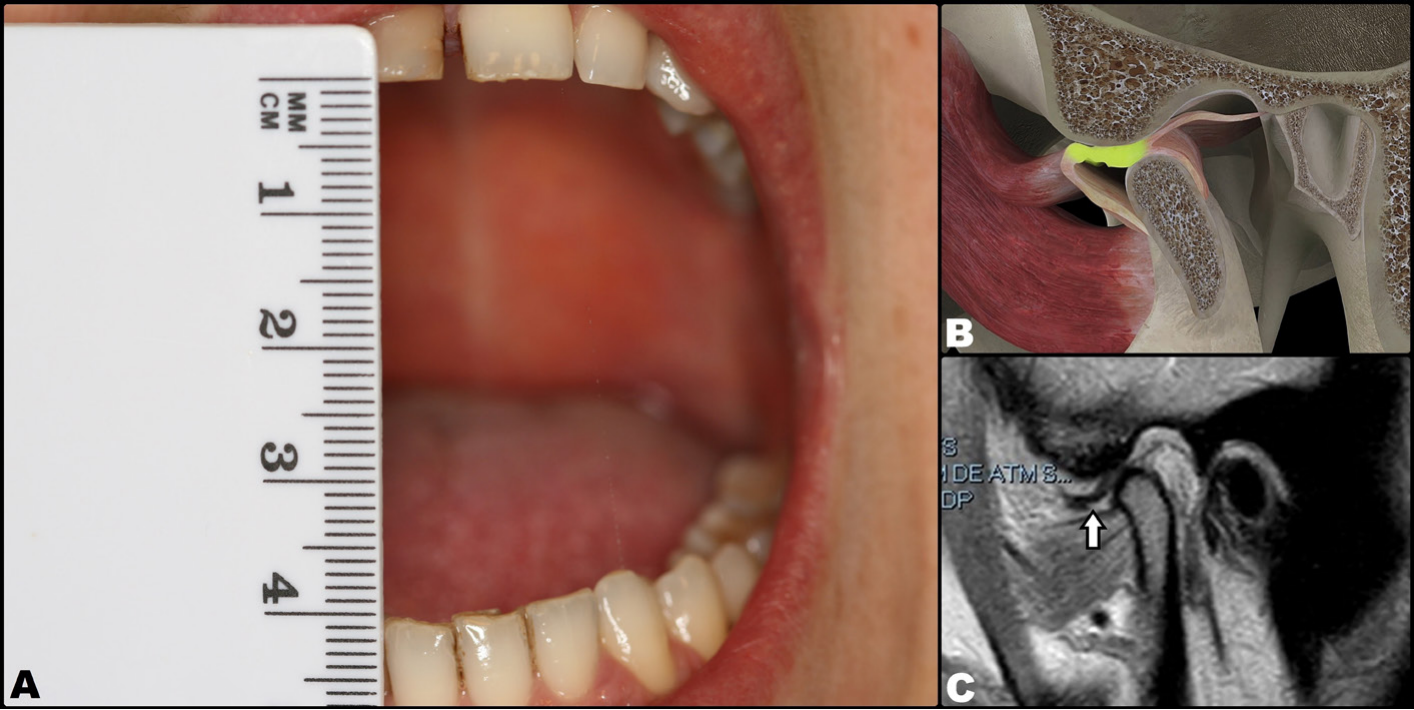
Final situation. Disc displacement without reduction and with no limited opening of the mouth of the patient. A: Correct opening reaching 40 mm. B: Three-dimensional representation at intra-articular level. C: Post-treatment magnetic resonance imaging view (arrow: disc displacement).
